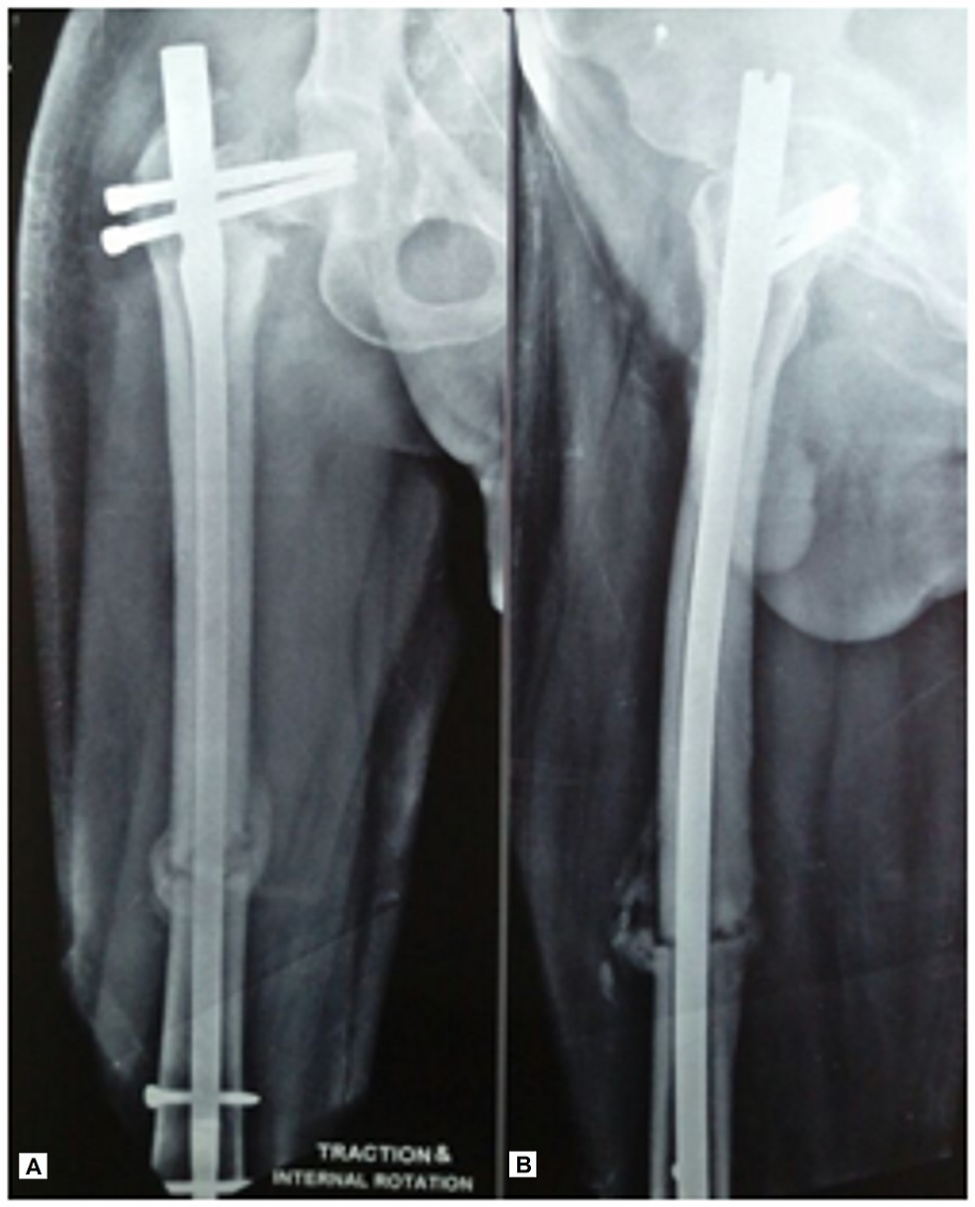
Radiographs of (A) the right hip and (B) thigh showing atrophic nonunion of the femoral neck with resorption of bone and varus malalignment along with hypertrophic callus with gap at the ipsilateral femoral shaft, on presentation.
